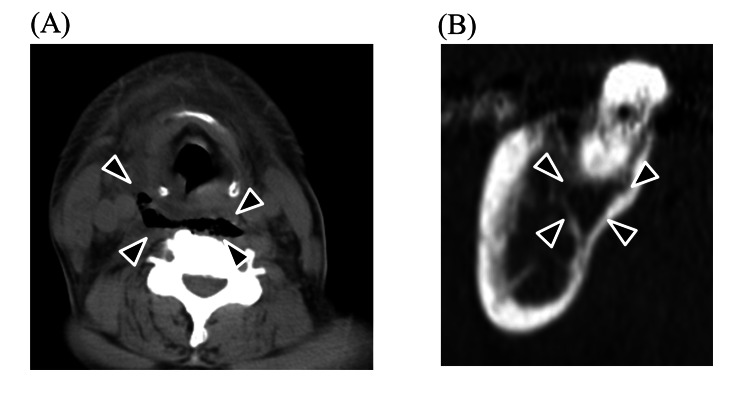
CT images at first visit of a patient (No.17) (A) Axial CT image. Black arrows indicate the part of a gas production in the retropharyngeal space.
